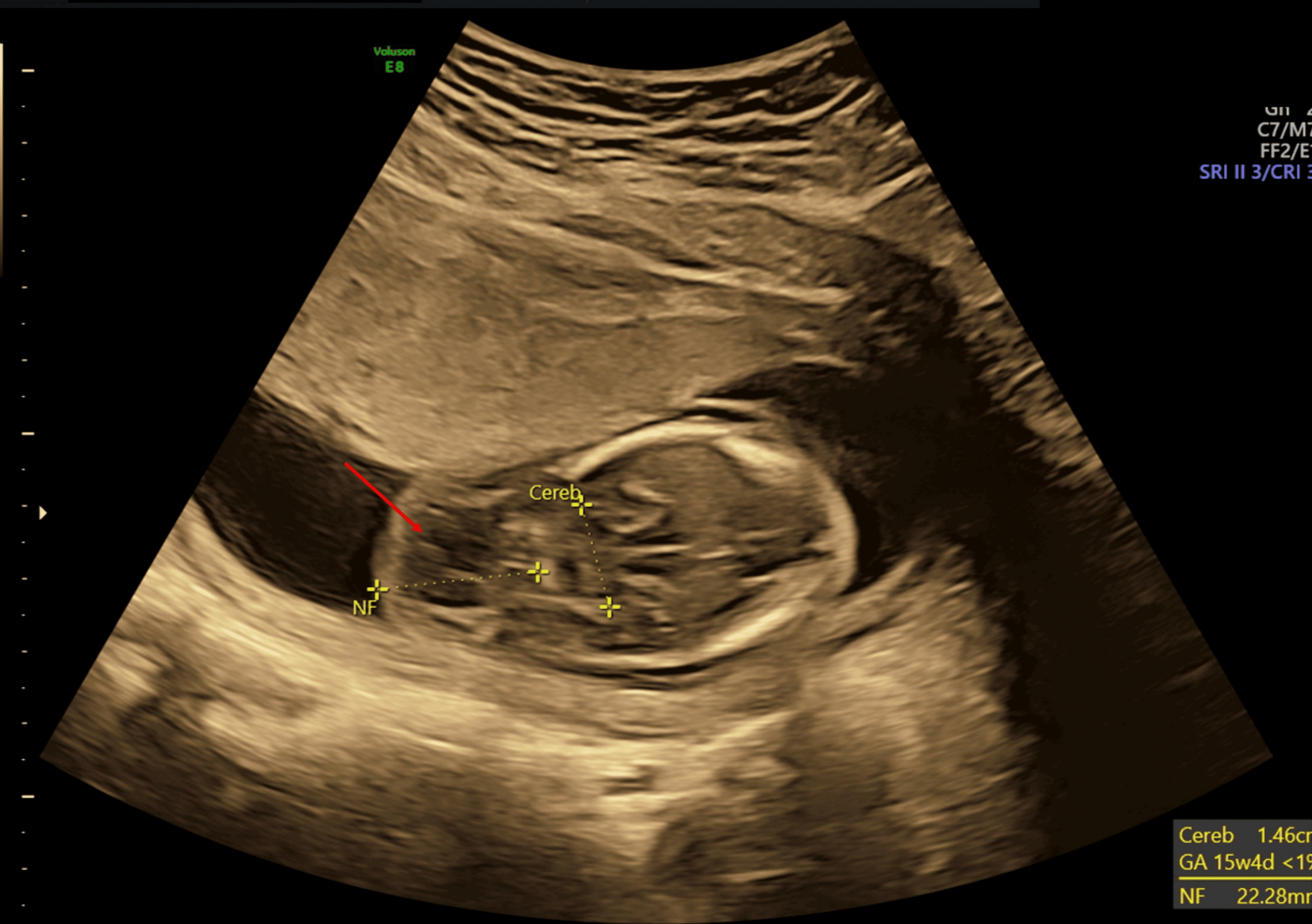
Antenatal ultrasound around the 17th week shows fetal hydrops and arthrogryposis; horizontal section of the fetal skull showing increased nuchal fold (red arrow).
